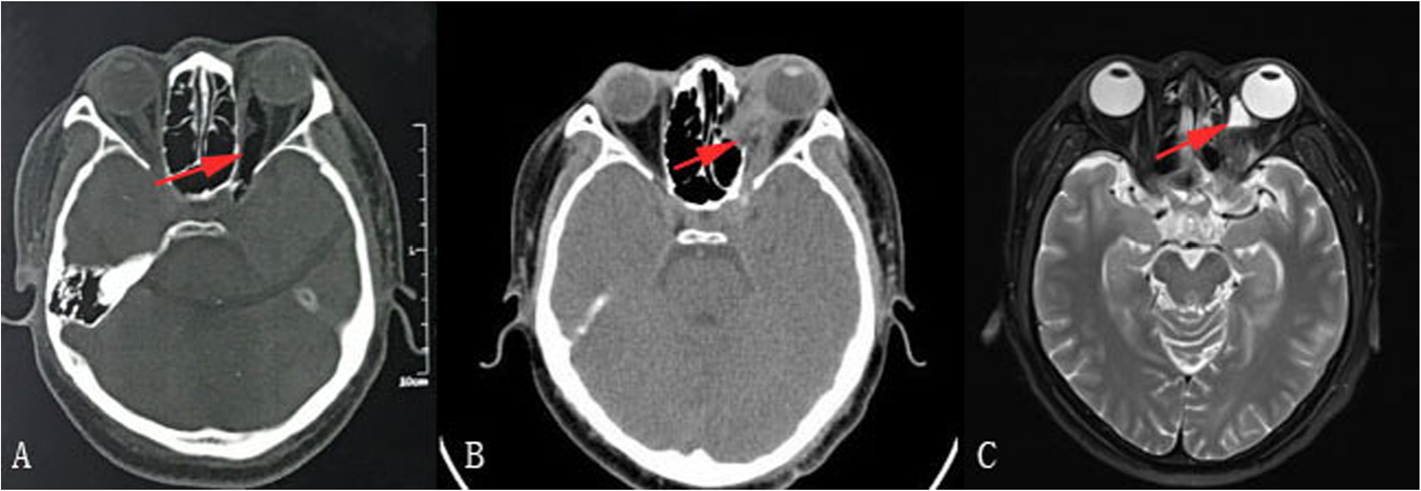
CT and MRI scans of the brain and orbit. a CT shows both orbit and brain lesions, as evidenced by decreased density in the orbit and brain. b CT shows an increase in the density in the orbit and a discontinuous medial wall of the orbital bone. c MRI sagittal T2 shows hyperintensity in the superonasal region of the left eye
